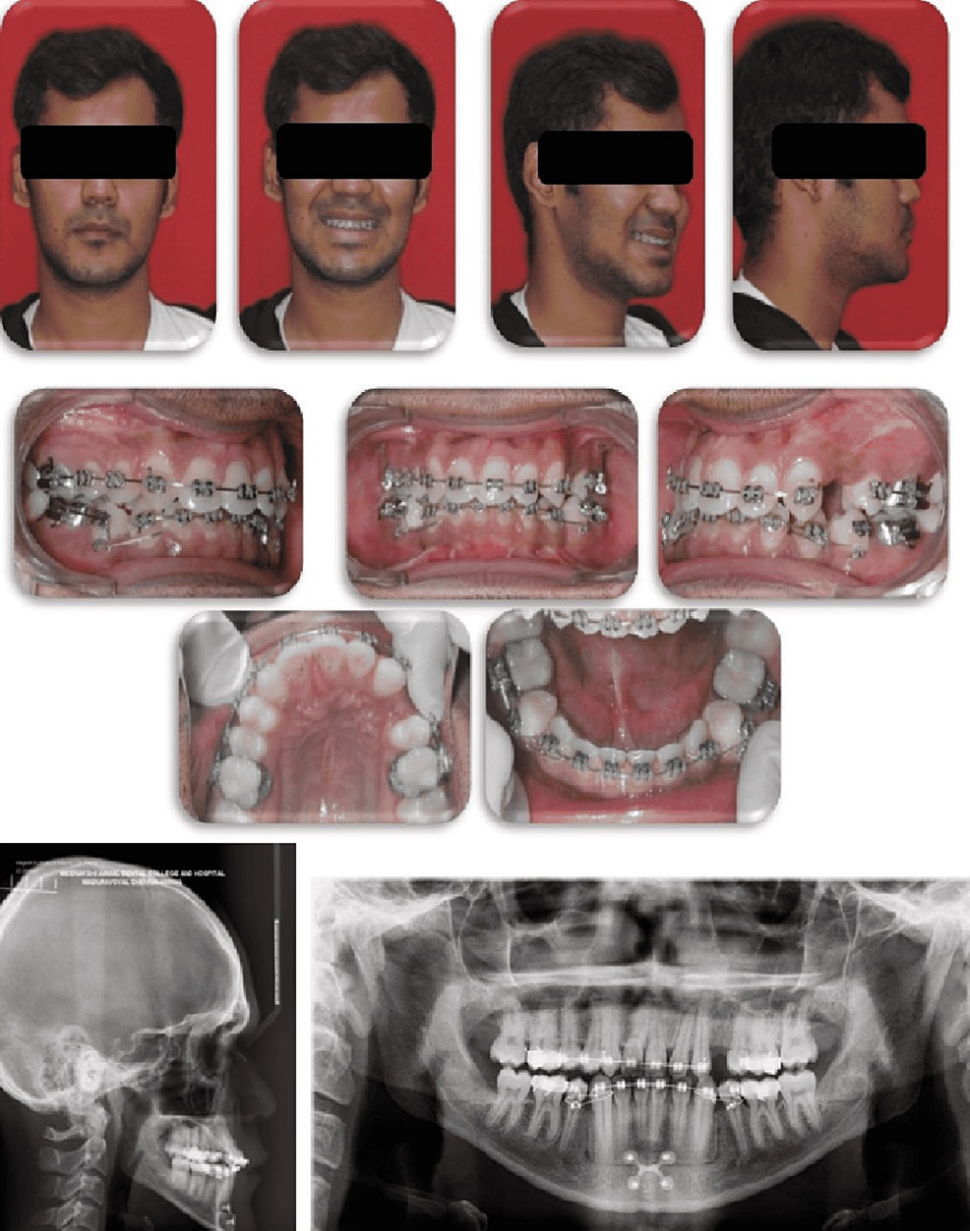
Post-surgery extraoral and intraoral photographs (lateral cephalogram and orthopantomogram)
